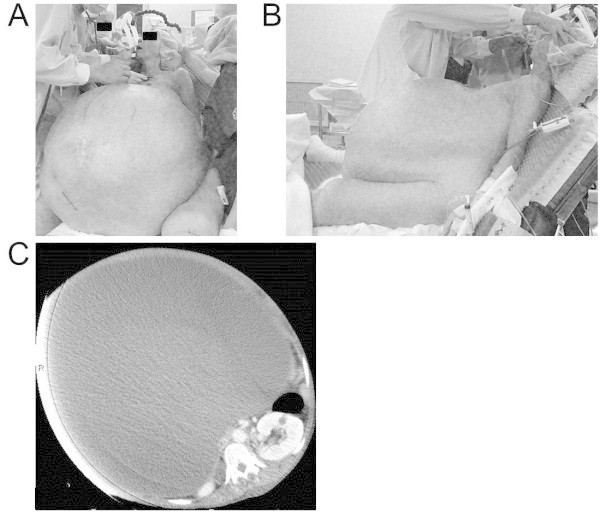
Preoperative frontal (A) and lateral (B) view of the patient showed remarkably distended abdomen with dilated subcutaneous veins. The abdominal computed tomography (C) of showed a huge cystic mass.
